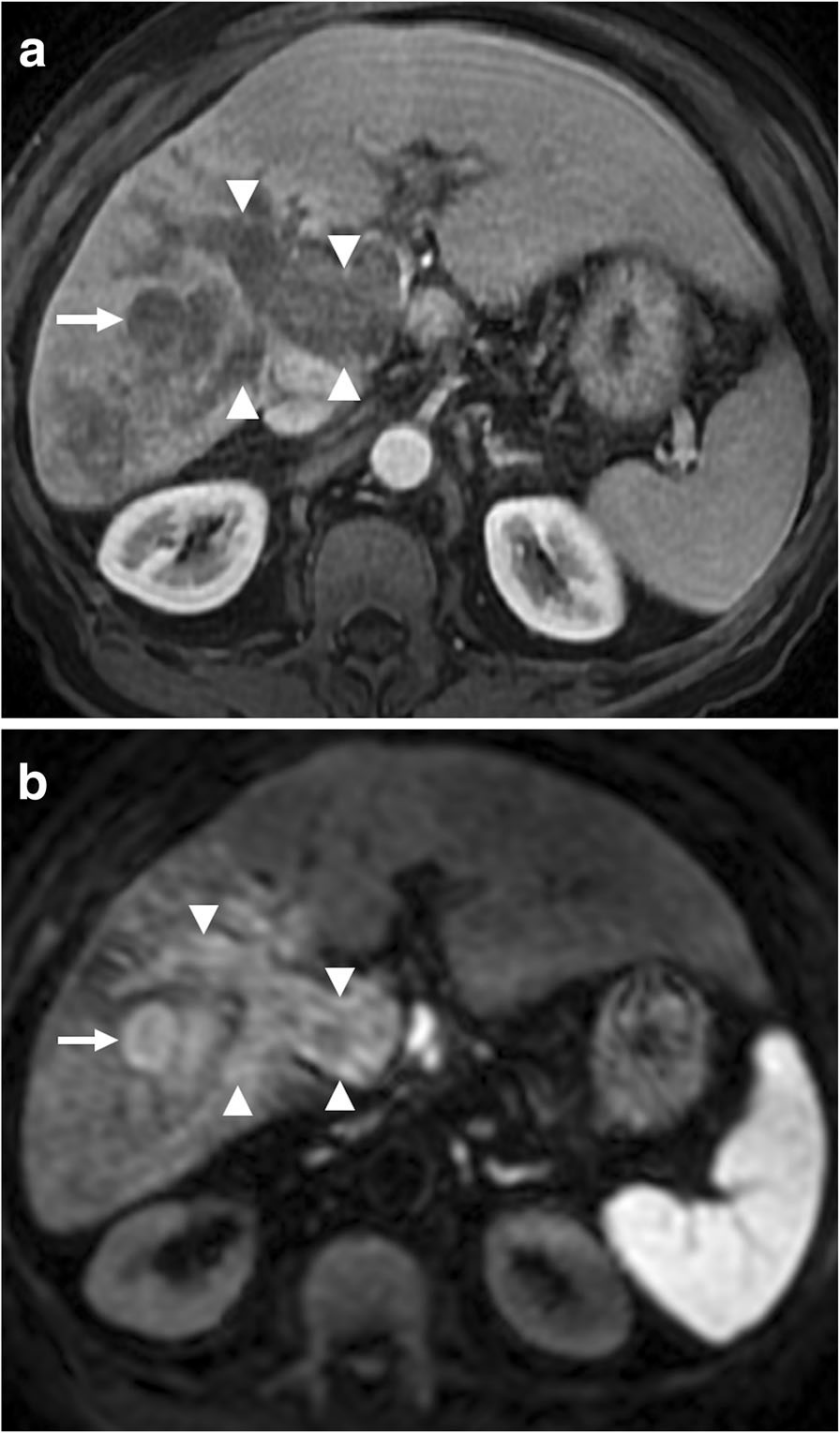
A 76-year-old male with known cirrhosis presents with elevated liver enzymes and alpha fetoprotein (AFP) levels. a Axial postcontrast T1W MR image demonstrates the HCC focus within the right liver lobe (arrow) with associating tumor of the main and right portal veins (arrowhead). Note contrast enhancement within the thrombus and its expansile nature extending outside the confines of the portal vein. b DWI sequence shows high signal intensity representing diffusion restriction of both the main tumor (arrow) and its intravenous component (arrowhead)
